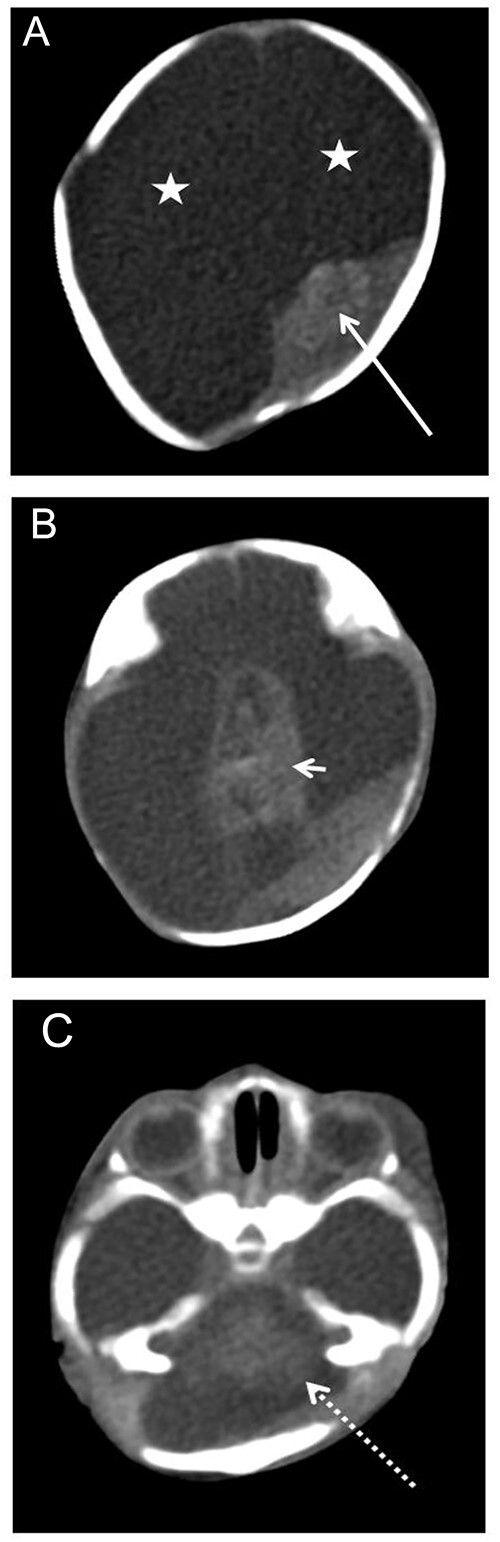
Axial brain postmortem computed tomography (PMCT) images: (A) brain parenchyma replaced by fluid (white stars), an ovoid dense mass instead of posterior part of the left brain parenchyma (long white arrow); (B) hyperdense central mass visible in the supra-chiasmatic region (short white arrow); (C) presence of different cerebellar structures (long dotted white arrow).
